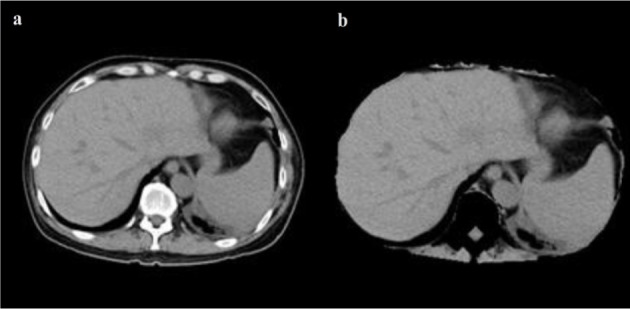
Original CT image (a), CT image after ribcage removal (b)
